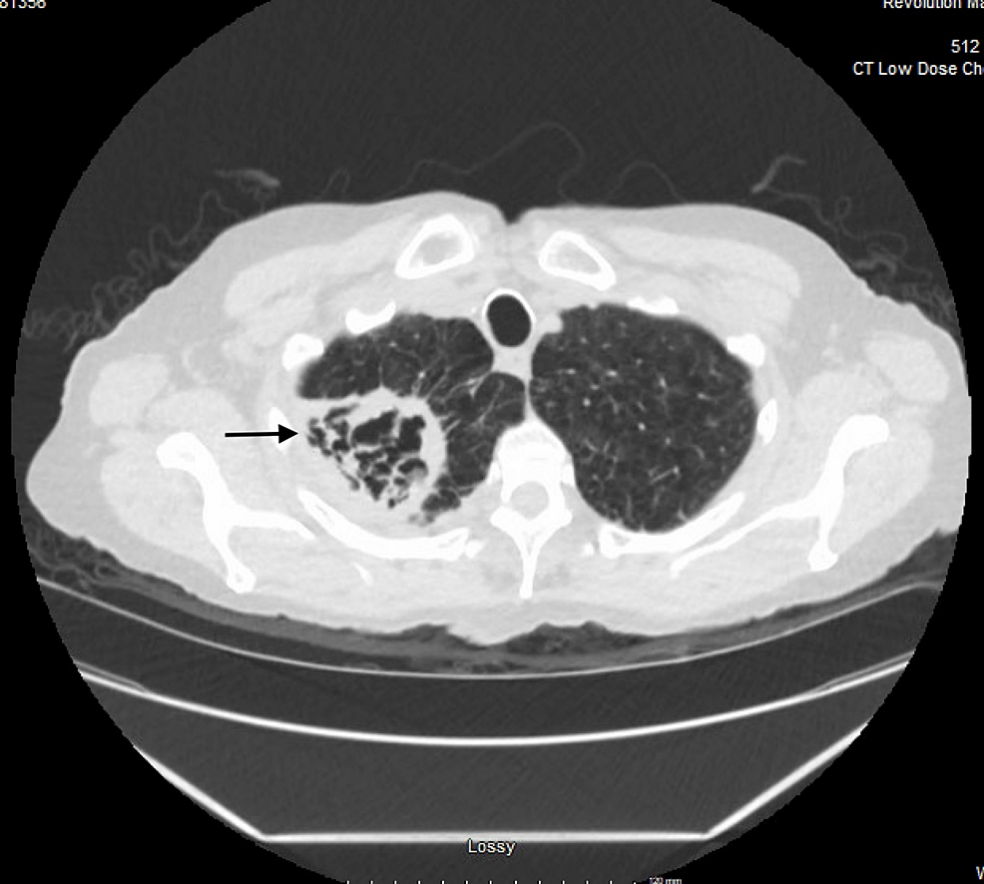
Axial view of Low dose CT chest showing a right apical cavitary lesion with heterogenous contents
